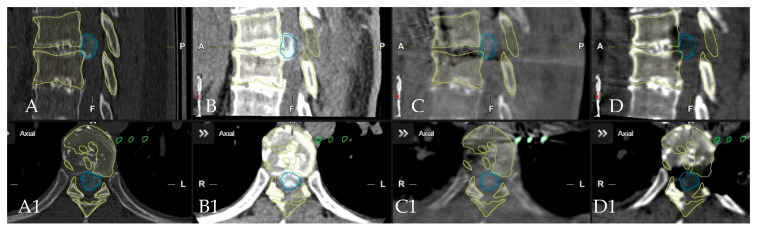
Patient No. 3, depiction of segmented vertebras in yellow and herniated disc in blue in preoperative (A,B) sagittal and (A1,B1). Axial CT with same segmented structure in intraoperative, control iCT scan following complete resection of the disc in (C,D) sagittal and (C1,D1) axial view.
